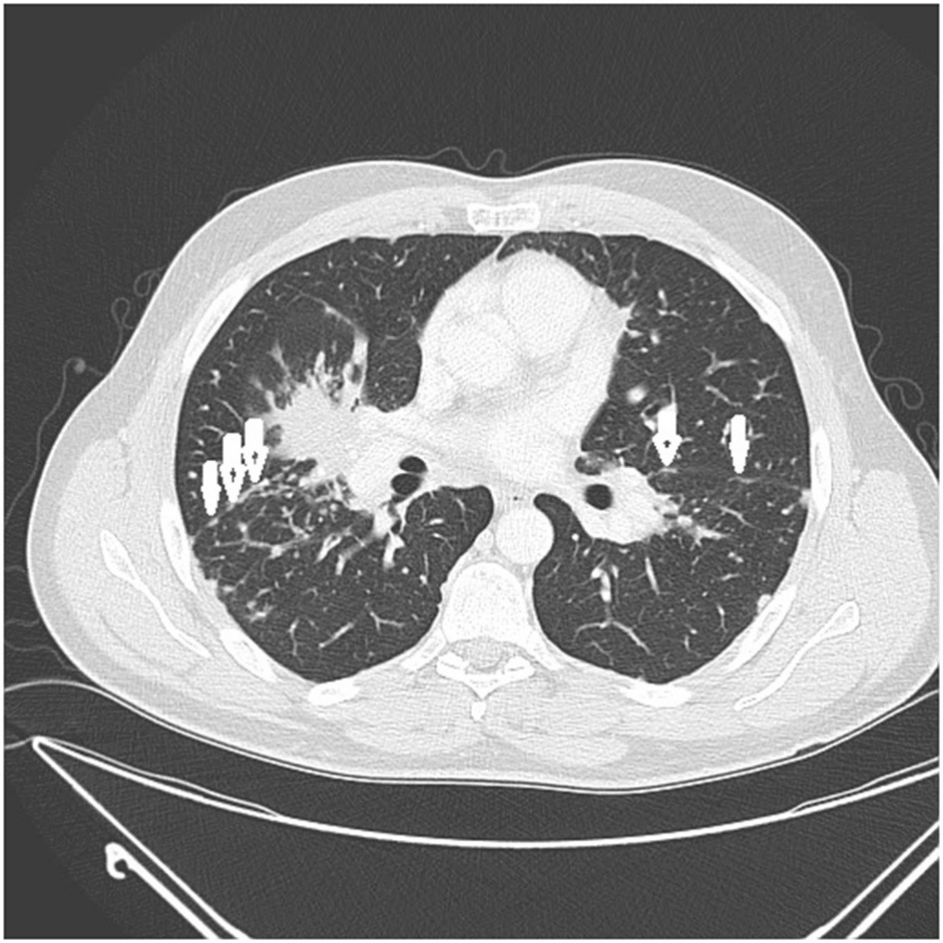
CT scan showing beading of the left and right major fissures with sarcoid granulomas (arrows).
